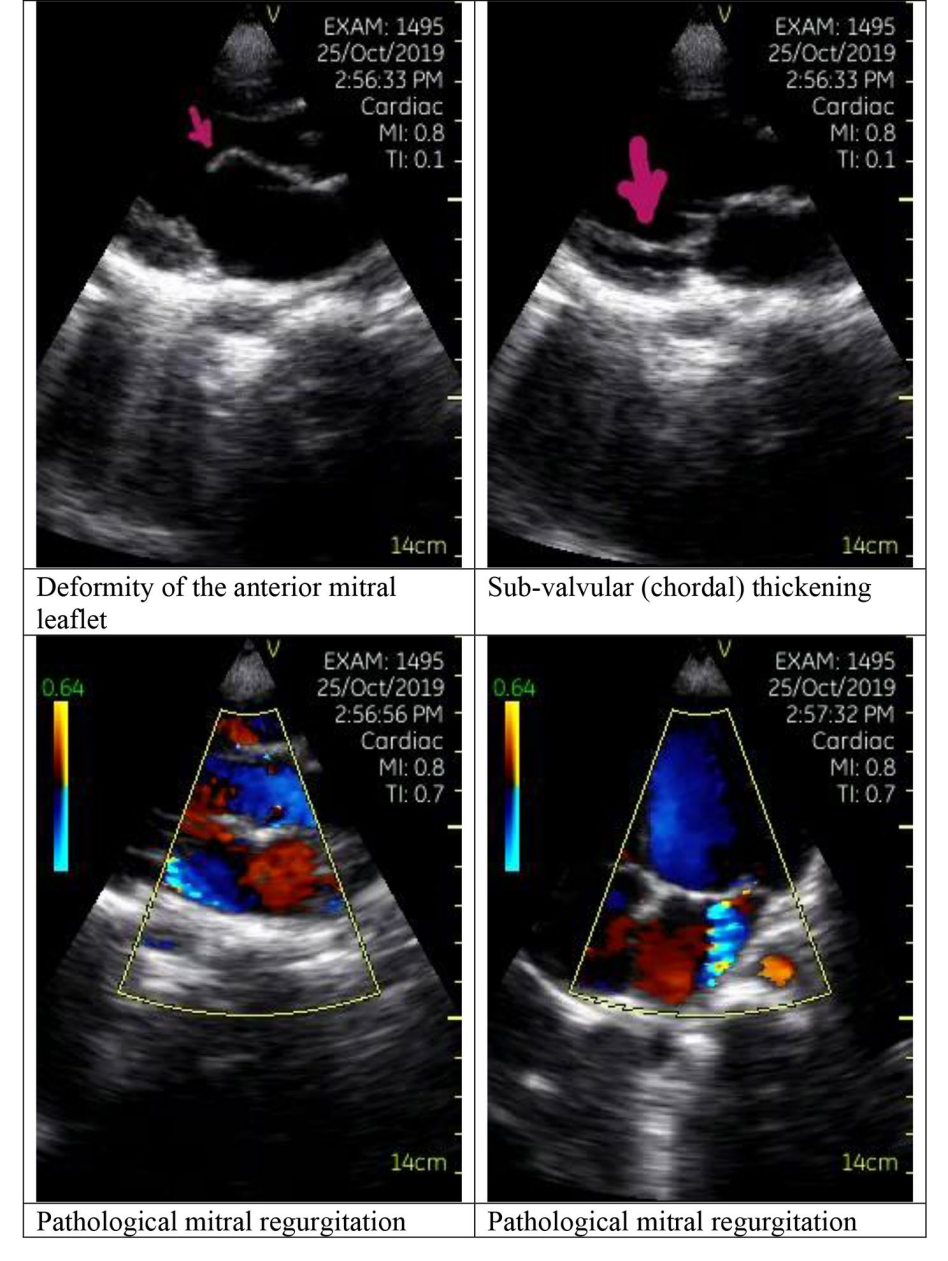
Echocardiographic findings in a child with definite RHD
